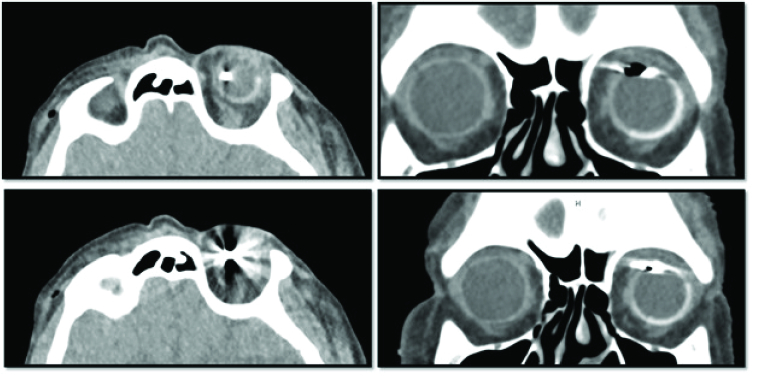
Orbital CT scan. Axial sections with suspected intraocular hyperdense metallic foreign body (Left panel). Large metallic foreign body with irregular borders complicated by streak artifact and air track superonasally embedding into the sclera (Right panel).
